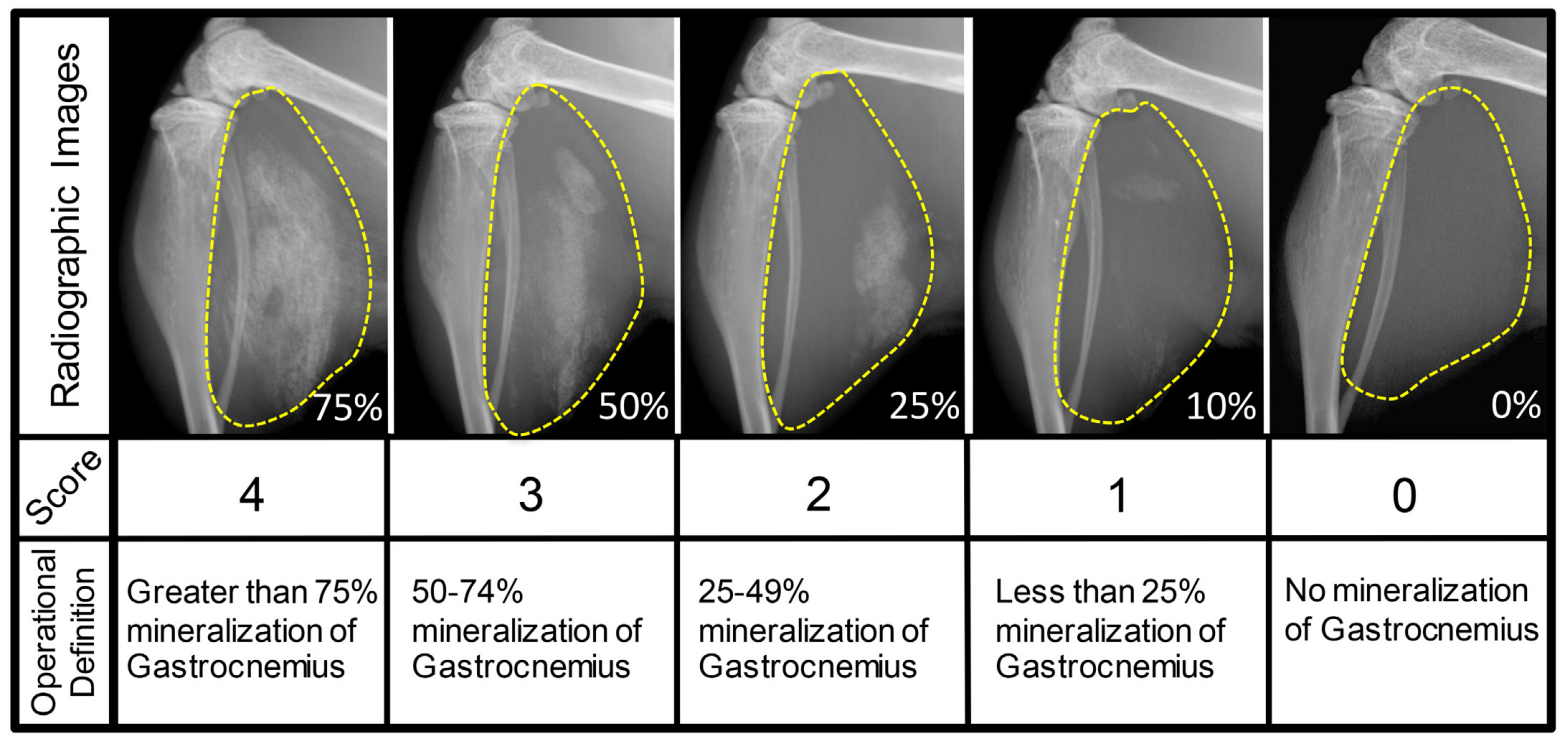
Soft Tissue Calcification Scoring System (STiCSS). STiCSS is an ordinal scale [0–4] developed for quantifying the varying degrees of soft tissue calcification from radiographic images of the lower extremity. Representative images of each STiCSS score are provided along with the operational definition designated to each score. Yellow dotted lines outline the area of interest for soft tissue calcification (the posterior compartment of the lower extremity), while the listed percentages correlate to the extent of soft tissue calcification within each sample image.
